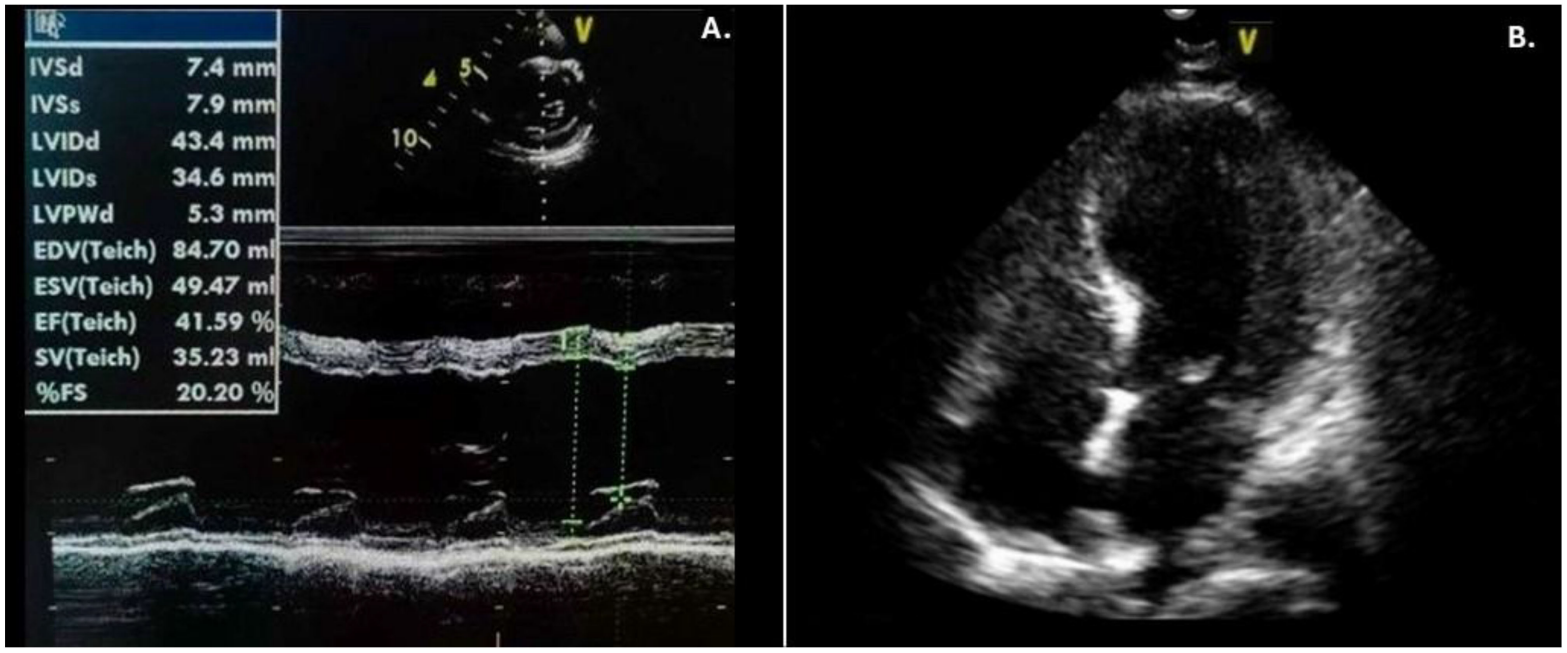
Echocardiography of our patient after resuscitation revealed left ventricular systolic impairment (A) with apical dyskinesia (B).
